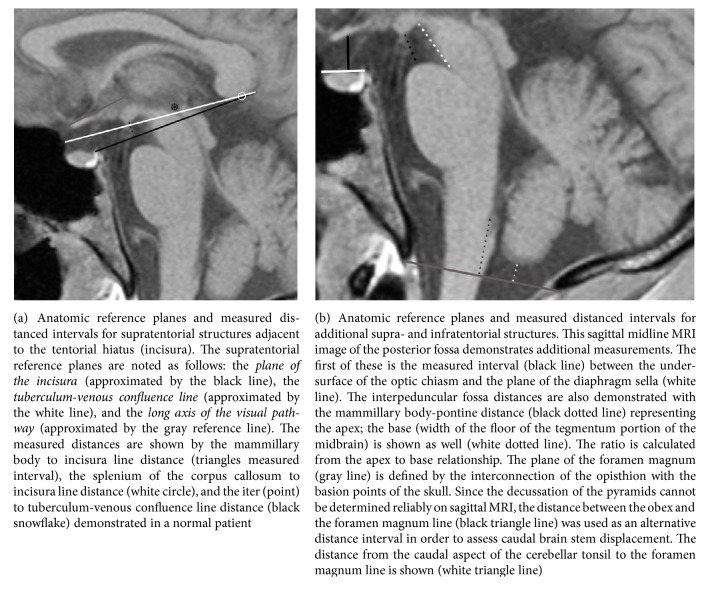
Incisural and foramen magnum related anatomic planes of reference and their MRI equivalent reference lines.* Technical note*. A symbol (rather than a line) has been used in the figures, when a measured distance is close to or equal to zero.
